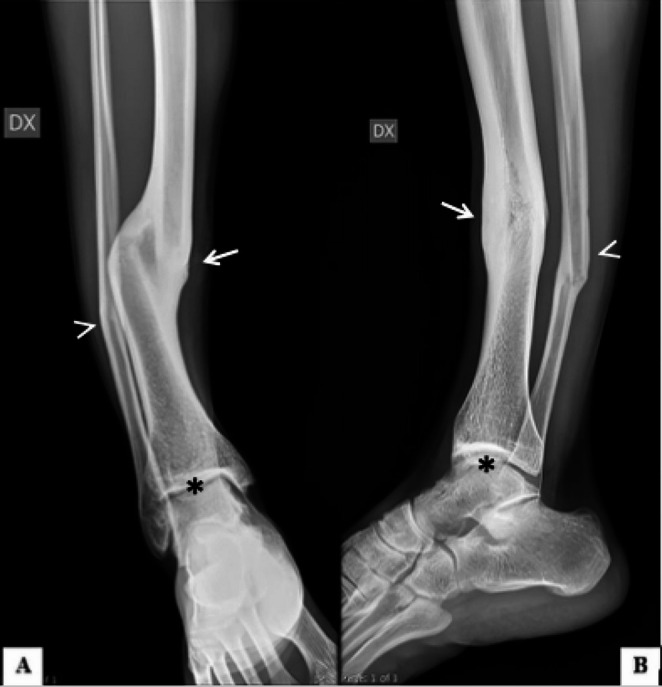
Victim of torture due to blunt force injuries in the lower limbs and signs of deformation. Leg x-ray in anteroposterior (A) and lateral (B) projections shows results of displaced fracture affecting the distal part of the right (DX) tibial (white arrow) and fibular diaphysis (white arrowhead) with residual structural deformity. Also note mild degenerative changes of the tibiotalar joint with reduction of the joint space and osteophytes (black asterisk)
